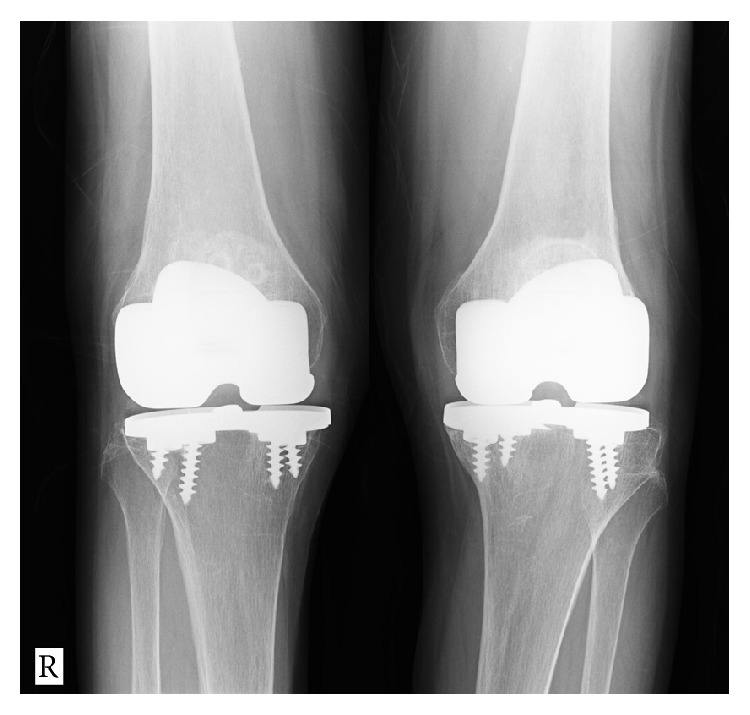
Follow-up plain radiograph obtained 15 years after surgery shows no change in the alignment of the right side and obvious deterioration of the varus malalignment (FTA of 190°) of the left side.
